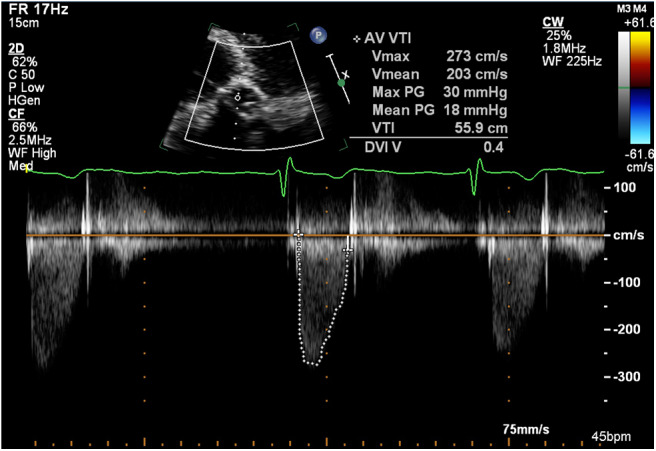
Transthoracic echocardiogram (January 2017): Continuous wave doppler in the apical 5 chamber view demonstrating a maximum transaortic velocity of 2.7 m/s.
